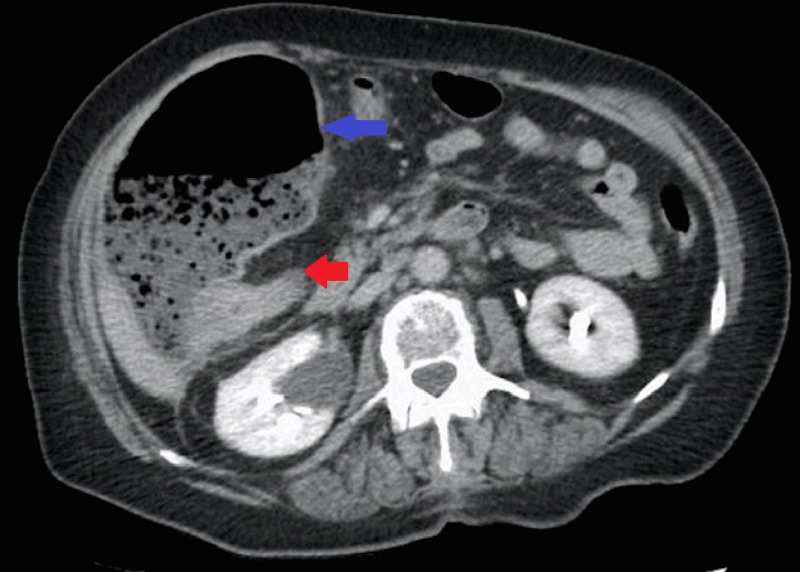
CT abdomen/pelvis Computed tomography (CT) of the abdomen and pelvis demonstrating a colonic mass (red arrow) with dilated colon (blue arrow) indicating obstruction.
